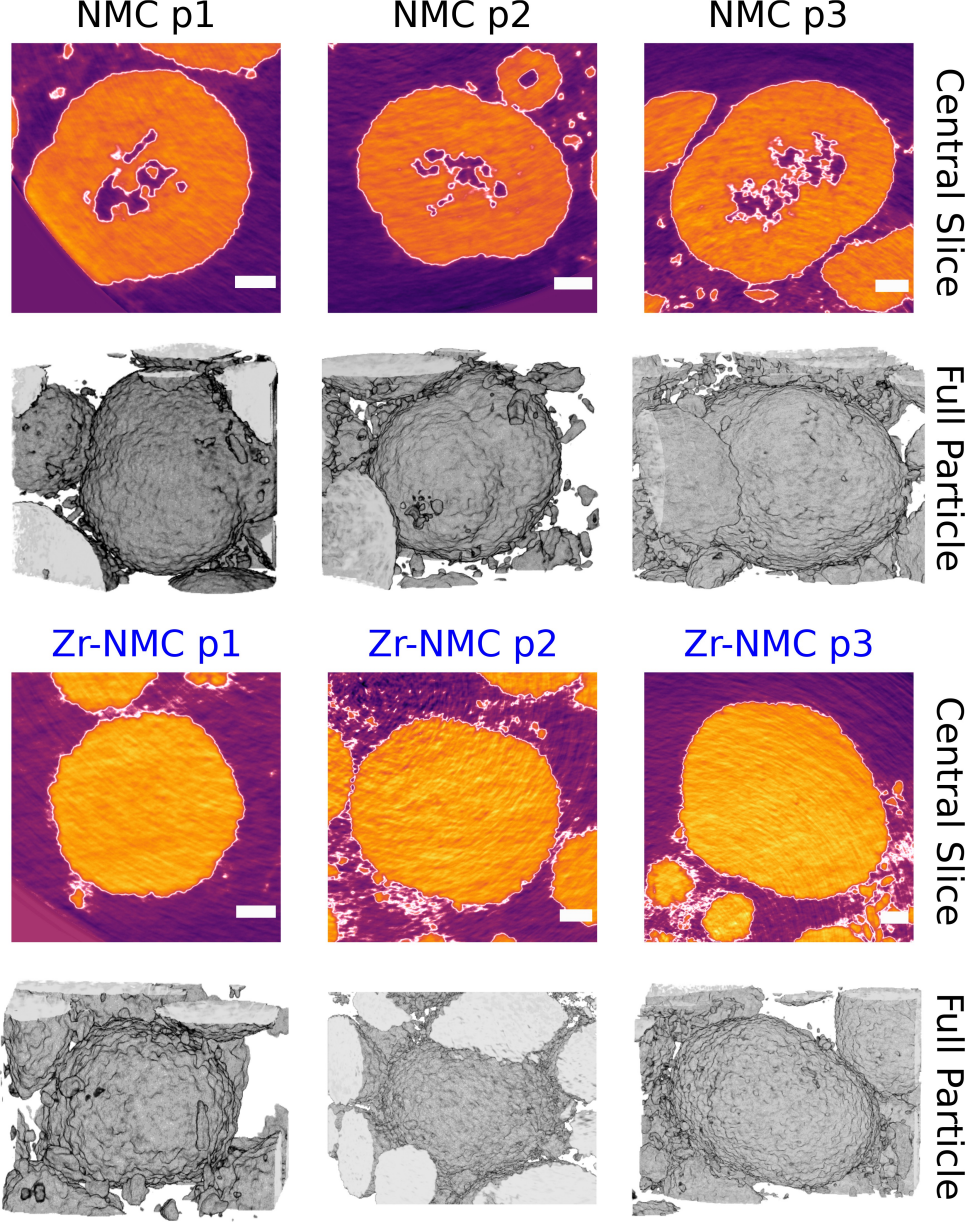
Nano‐CT measurements of three different undoped NMC811 and Zr‐NMC811 secondary particles. The 1st and 3rd rows show the 2D cross section slice inside the particles core for undoped NMC811 and Zr‐NMC811 respectively. The 2nd and 4th rows show the 3D rendering of the outer shape of the selected particles. In the 1st and 2nd rows, the scale bar size is 2 μm.
